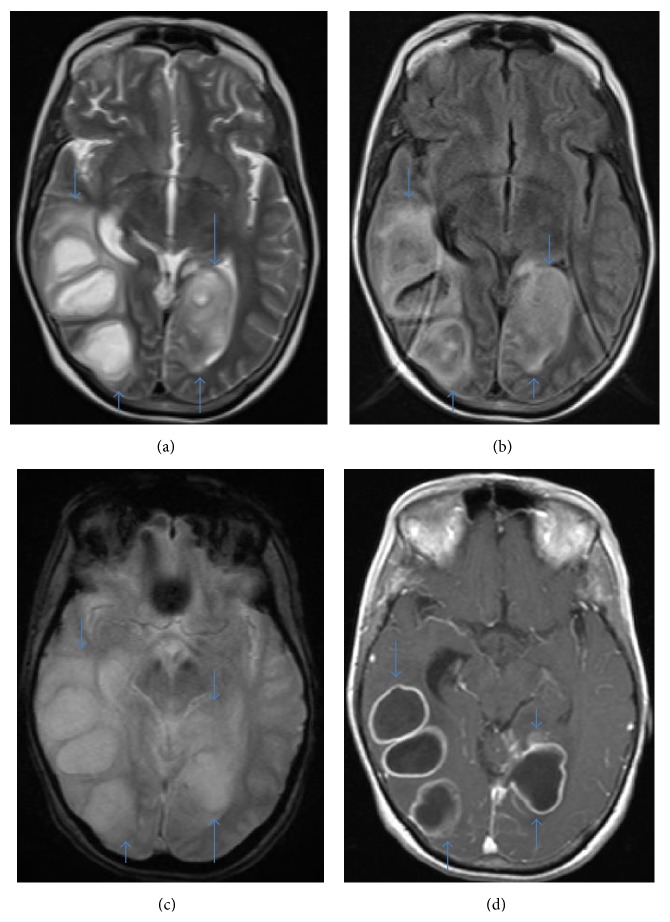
(a) Axial T2 weighted image, (b) axial FLAIR weighted image, (c) axial GRE weighted image, and (d) axial T1 weighted postcontrast image show bilateral temporal-occipital lobe abscesses (arrows).
